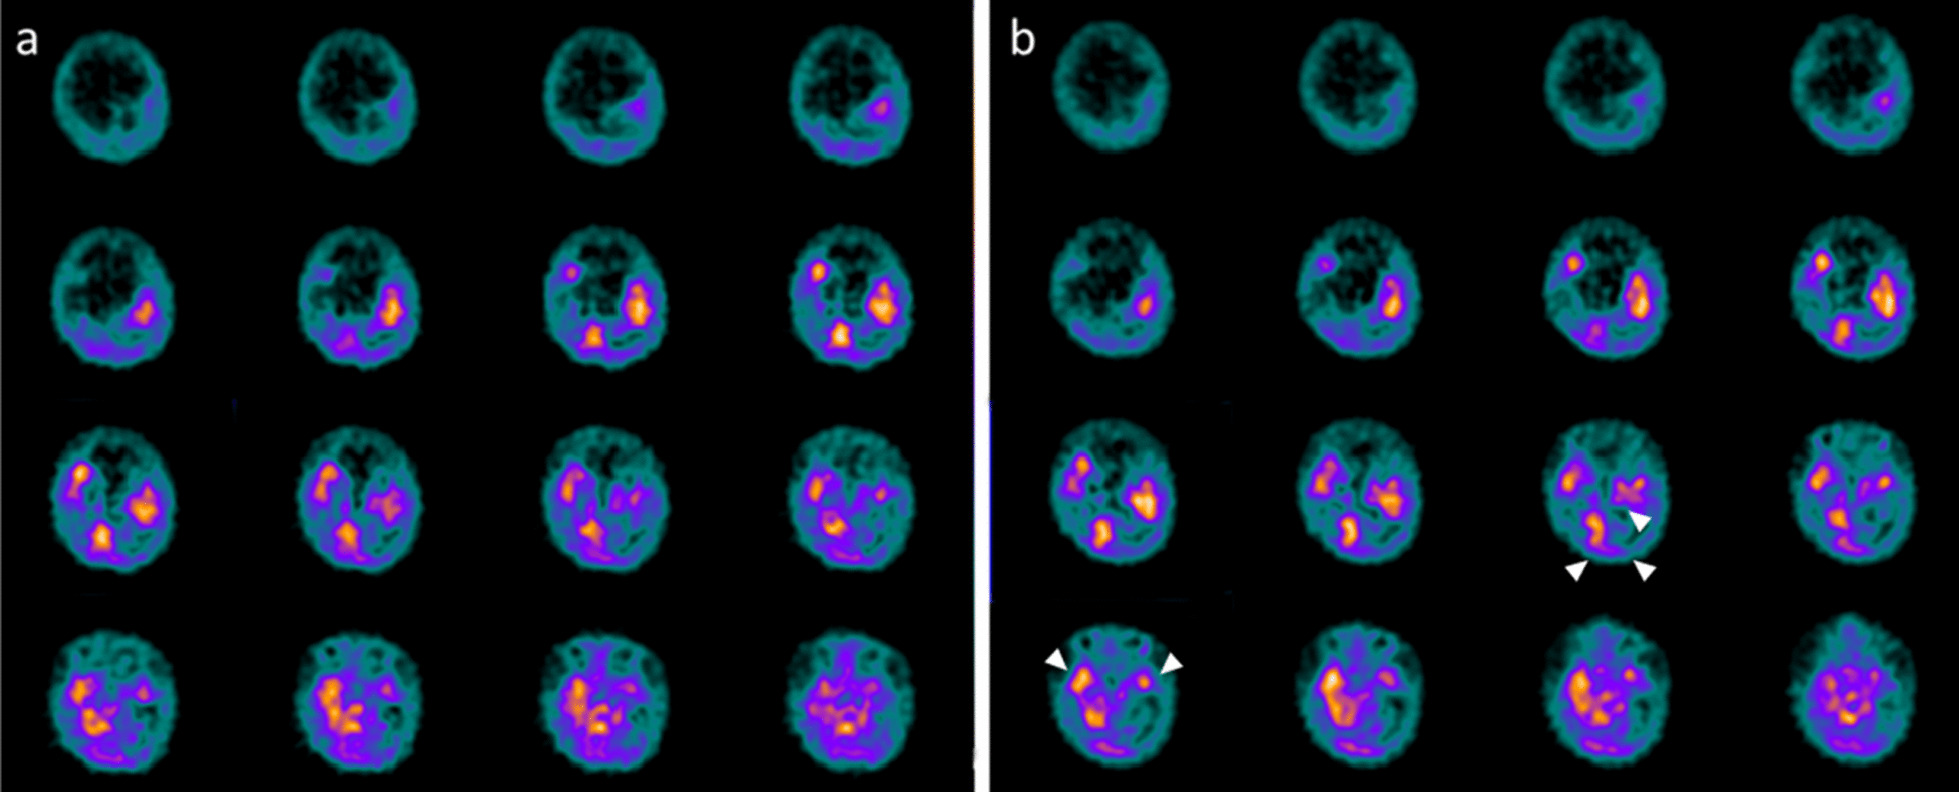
SPECT before and after the treatment with hr-NGF. a, b: Perfusion SPECT images before (a) and after b hr-NGF administration. ^99m^Tc-HMPAO SPECT images (transaxial slices) before hr-NGF treatment showed a severe reduction in radiotracer uptake (hypoperfusion) in almost all cortical and subcortical areas as well as in the cerebellum (a). After hr-NGF treatment, an increase in ^99m^Tc-HMPAO uptake was found in the left temporal cortex (+ 20%), right anterior temporal cortex (+ 13%), left thalamus (+ 10%) and bilateral occipital cortex (right: + 14%; left: + 18%, respectively) (b)
